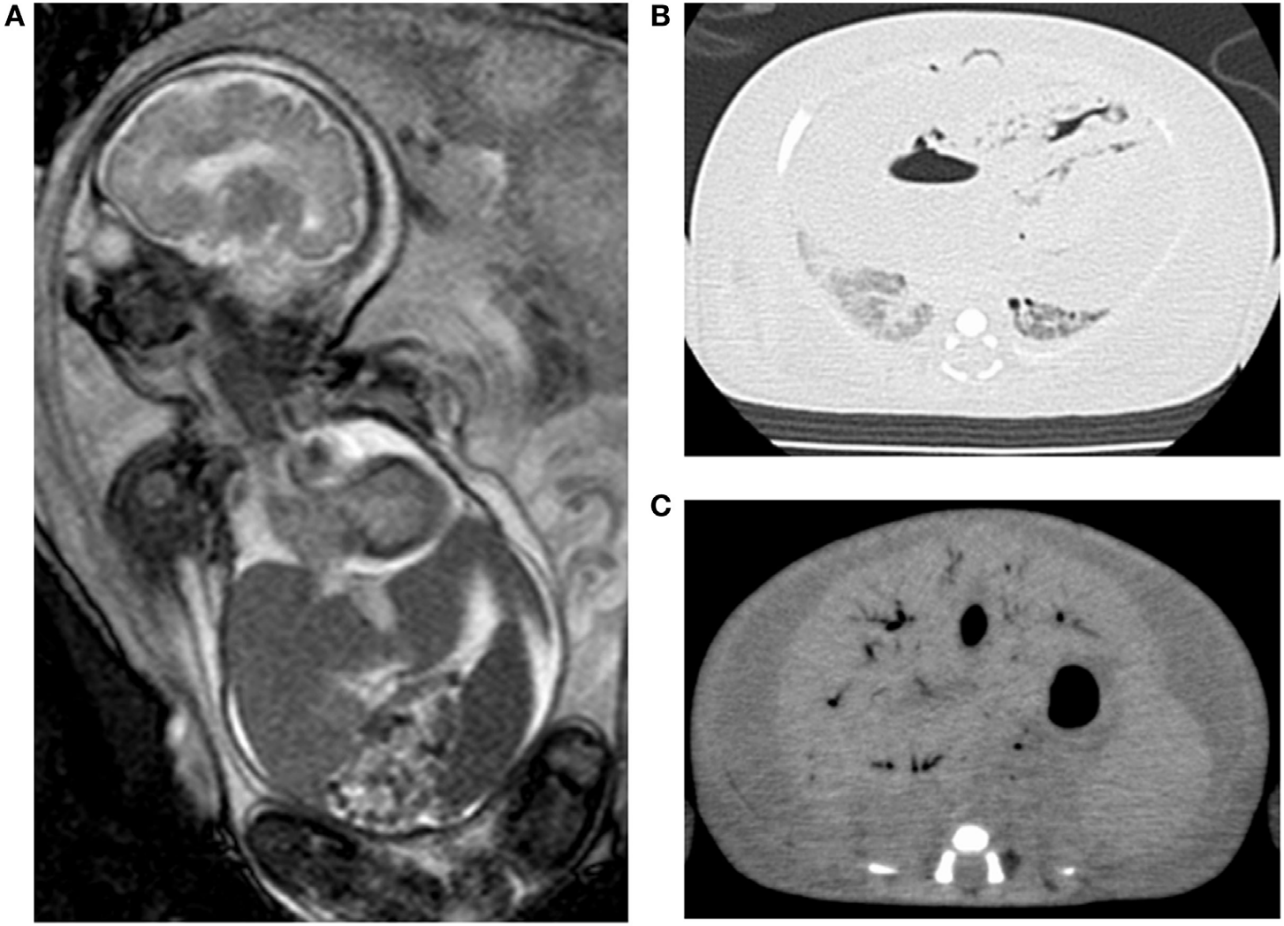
Images of case 2. Fetal magnetic resonance imaging shows massive ascites, hepatomegaly, and compressed lungs (A). Postmortem chest (B) and abdominal CT (C) show pulmonary hypoplasia and massive ascites with hepatomegaly.
